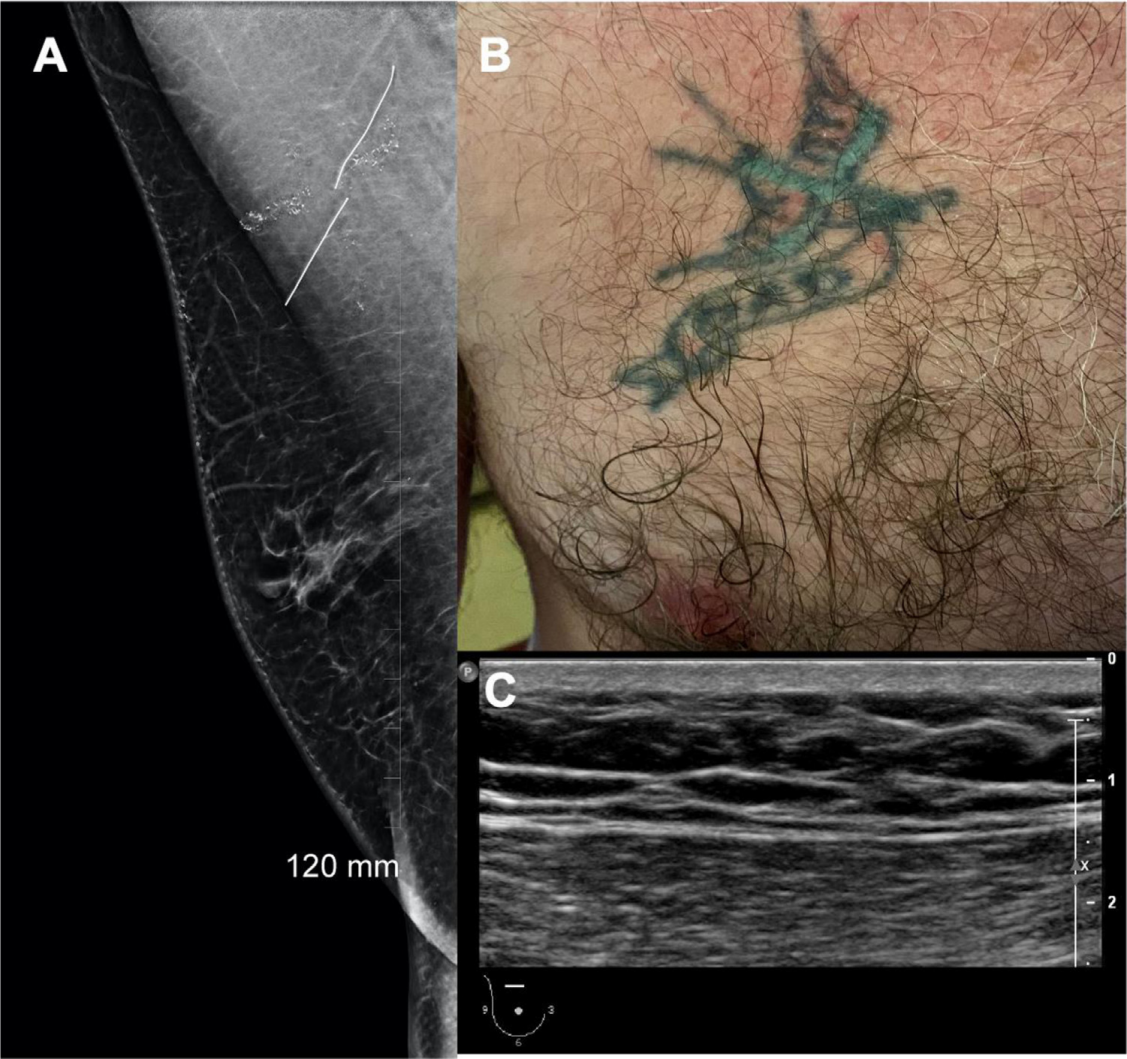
A. Slice 53/74 in the medial breast from the digital tomogram demonstrating the ‘BB’ marker directly overlying the calcifications. B. The corresponding color tattoo. C. Normal ultrasound of the superomedial right breast.
